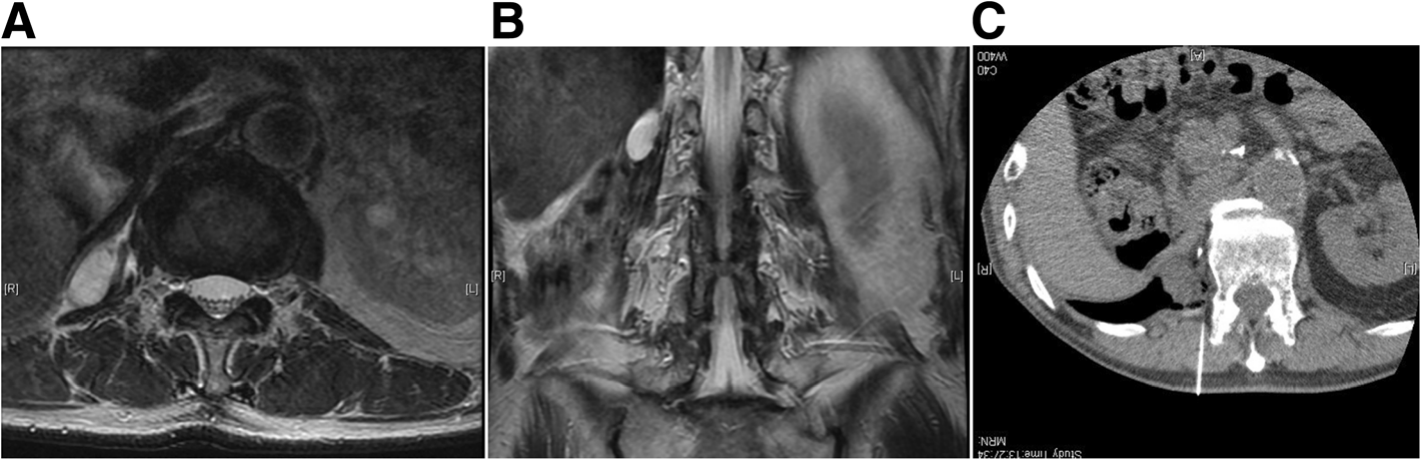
Locally recurrent, 3 cm, dedifferentiated liposarcoma in the retroperitoneal paravertebral region 1 year after initial surgery (a, b). c Under CT guidance, air was transperitoneally infused to displace the bowel just adjacent to the recurrent tumor
